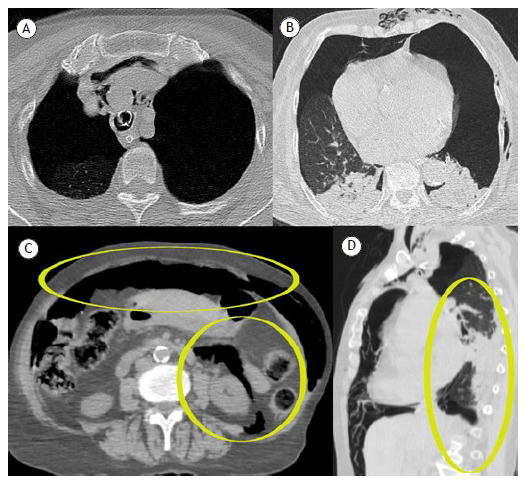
Pneumomediastinum (A) and large bilateral pneumothorax and emphysema on chest CT (B). Pneumoperitoneum and retroperitoneum on abdominal CT scan ellipses (C), and gas dissection of the thorax and infradiaphragmatic gas on sagittal CT slice (D).
